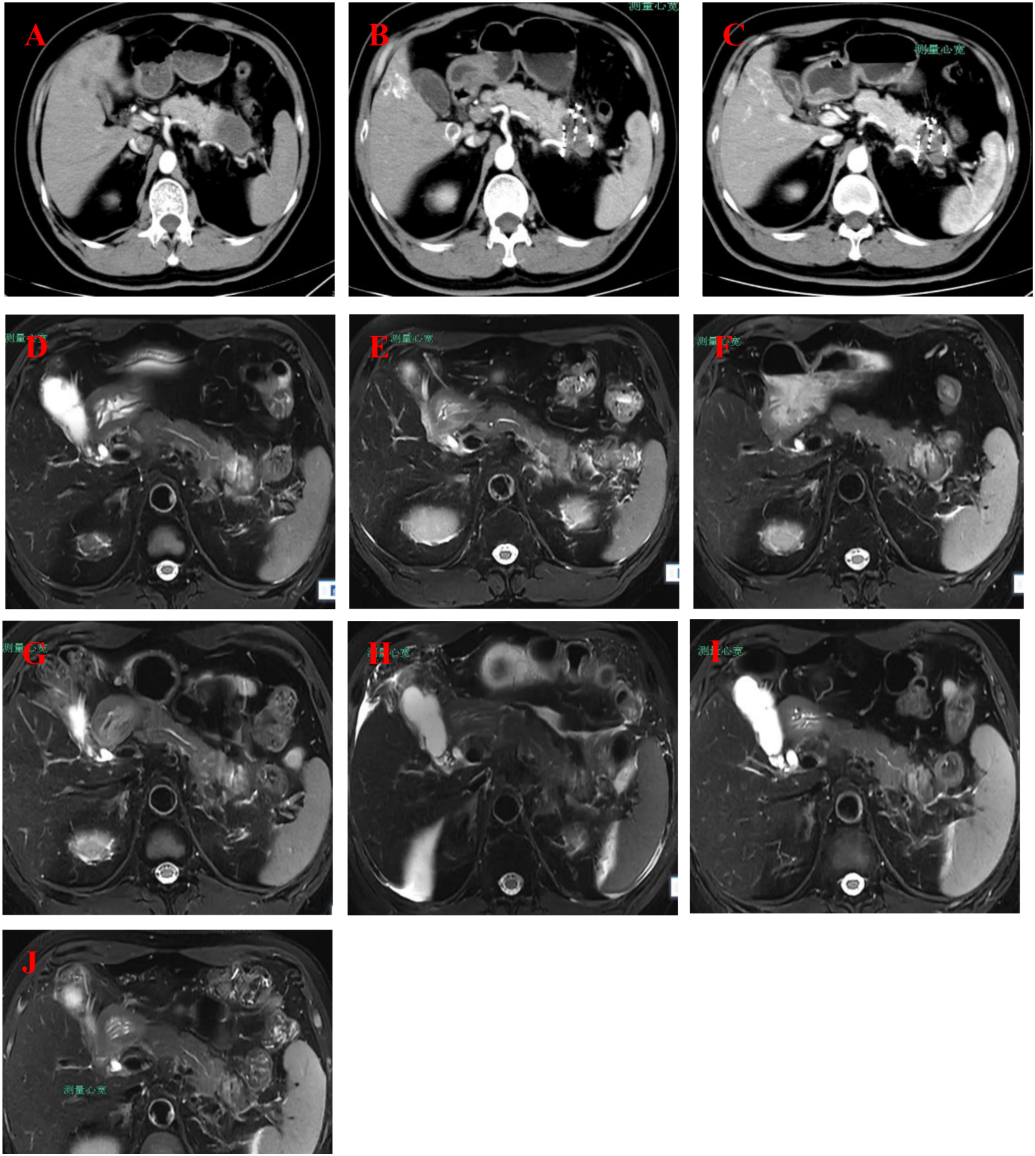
Medical imaging progress of pancreatic and liver conditions. (A) (2024-4-4): CT scan of the abdomen showing pancreatic tail mass, suspected as a malignant tumor, with liver metastasis and enlarged lymph nodes. (B) (2024-5-13): MRI scan of the abdomen depicting a pancreatic tail tumor, with signs of liver metastasis, gallbladder inflammation, and possible kidney involvement. (C) (2024-6-11): CT and MRI showing unchanged pancreatic mass with metastatic liver lesions, mild gallbladder inflammation, and lymphadenopathy. (D) (2024-8-21): MRI scan highlighting the pancreatic mass, possible splenic vein thrombosis, and multiple liver lesions showing no significant change. (E) (2024-10-9): MRI of the abdomen showing a stable pancreatic mass with continued liver metastasis and a possible inflammatory process in the kidneys. (F) (2024-11-25): MRI scan showing pancreatic tumor with minimal changes, continued liver metastases, and moderate kidney abnormalities. (G) (2024-12-16): MRI showing unchanged pancreatic tumor, suspected splenic vein involvement, and stable liver lesions with mild gallbladder inflammation. (H) (2025-1-13): MRI showing slight growth in pancreatic tumor, stable liver metastases, and increased abdominal fluid accumulation. (I) (2025-2-5): MRI depicting stable pancreatic mass, unchanged liver metastases, and decreased pleural fluid. (J) (2025-2-26): MRI showing slight reduction in the edge enhancement of the pancreatic tumor, with stable liver metastases and mild pleural effusion.
